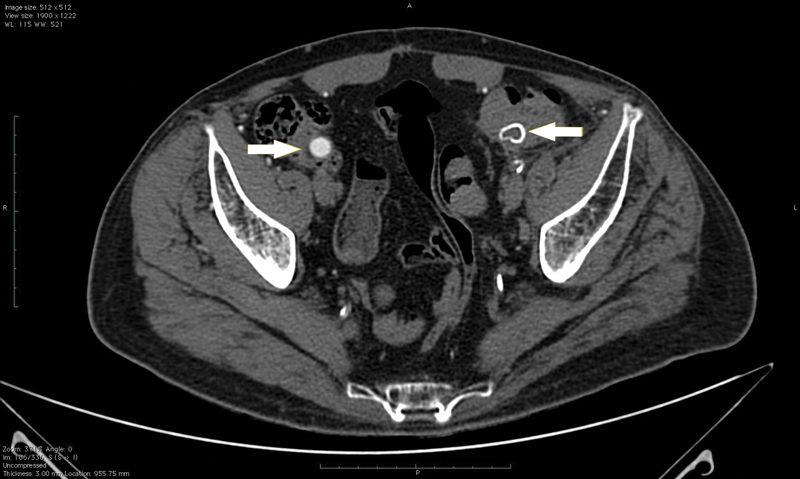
Preoperative computed tomography scan. Axial image indicating that the thrombosed left and right limbs of the graft (shown by white arrows) have an intraluminal course through the sigmoid and cecum, respectively.
